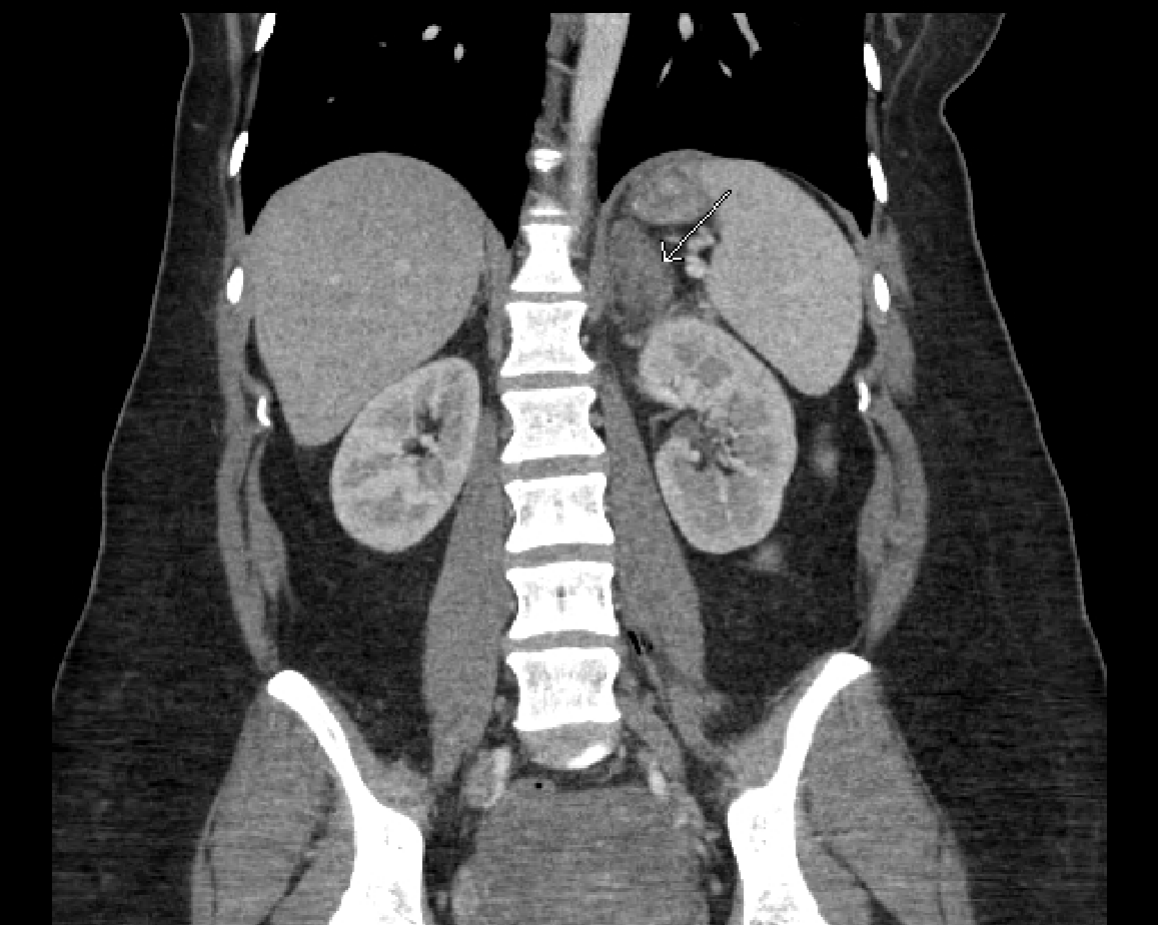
Sagittal CT scan image demonstrates left adrenal hyperattenuation (arrow) consistent with adrenal hemorrhage
